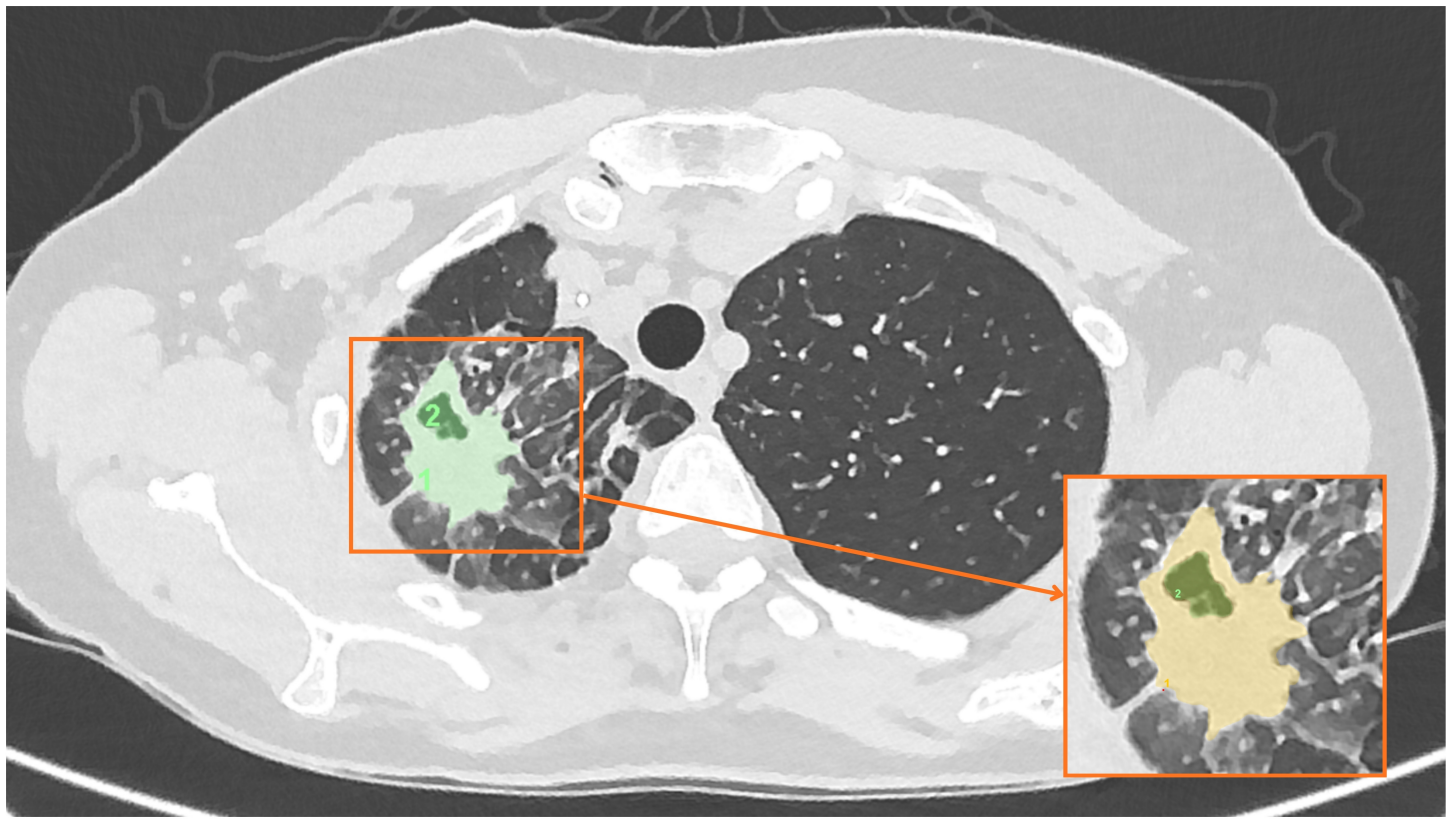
Baseline low-dose CT chest at Day 0 (initiation of nebulized PRP treatment) demonstrating persistent pulmonary consolidation without interval resolution.
